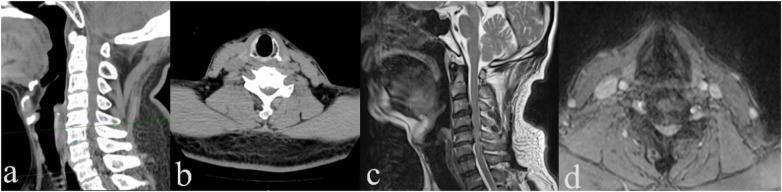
Preoperative cervical spine CT and MRI. (a) Cervical spine CT sagittal view; (b) cervical spine CT axial view at C5/6; (c) cervical spine MRI sagittal view; and (d) cervical spine MRI axial view at C5/6.
